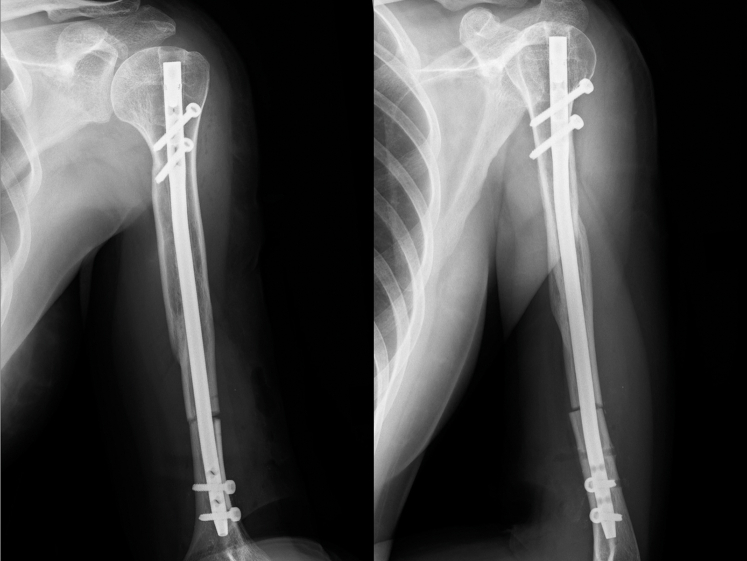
Plain radiographs after humeral rotational osteotomy.
